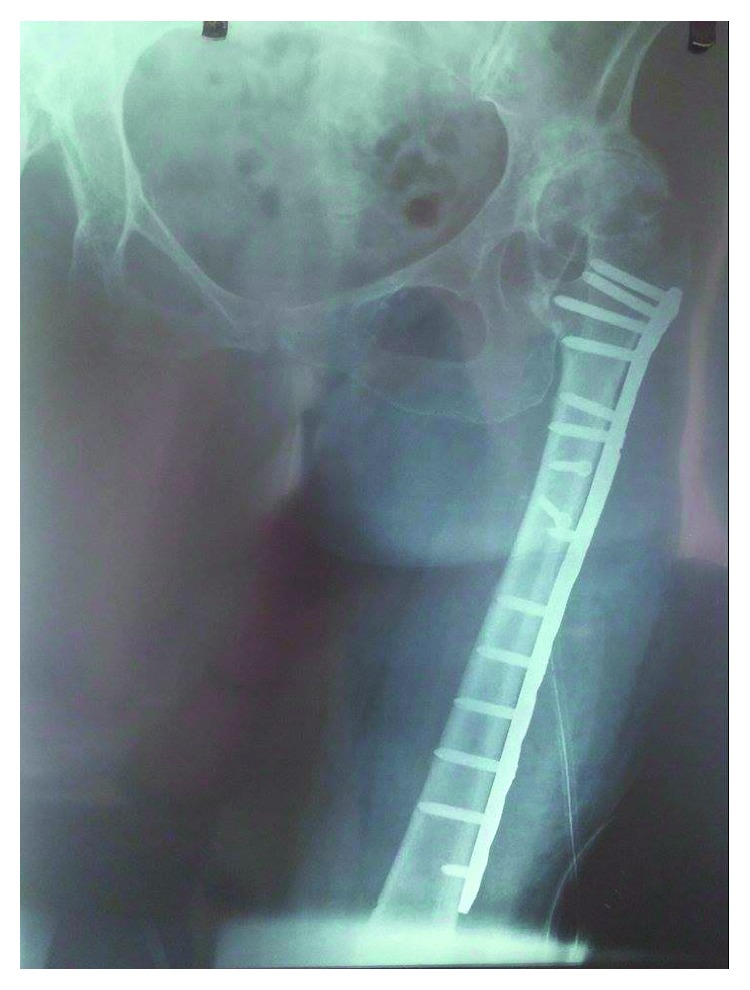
Anteroposterior radiograph of the left femur after ORIF (POD 1).
